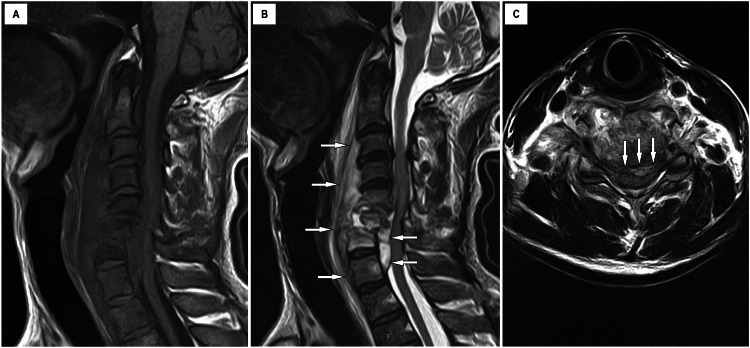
Preoperative cervical plain MRI (A) Sagittal view of cervical T1-weighted MRI revealed erosion and collapse of the C5, C6, and C7 vertebral bodies. (B) Sagittal view of cervical T2-weighted MRI. Arrows indicate epidural abscess from C3 to Th1. (C) Axial view of cervical T2-weighted MRI at the C6 level revealed severe spinal cord compression due to massive epidural abscess and collapsed vertebral bodies. Arrows indicate epidural abscess at the C6 level.
